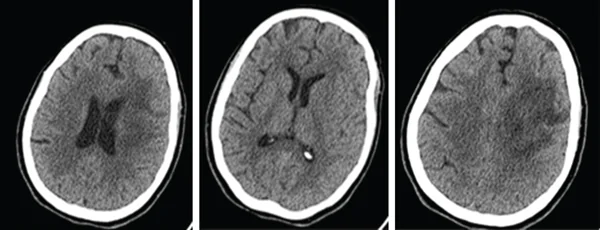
Head CT scan before discharge with normal evolution of the cerebral hemorrhage, resolution of midline shift, and re‐expansion of the left ventricle.
